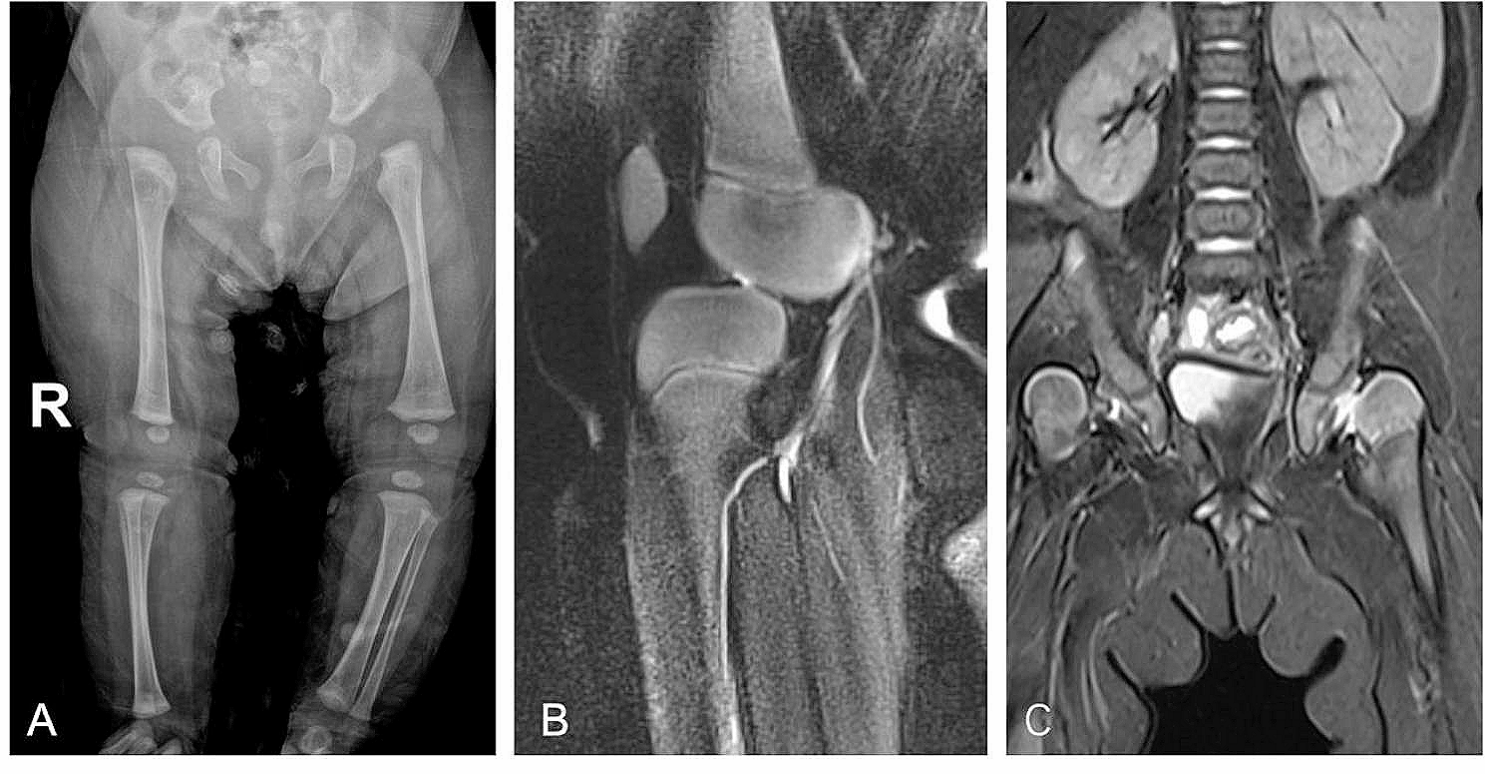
The full-length X-ray of the lower extremity revealed subluxation of the right knee joint, anterior shifting of the tibia, and external rotation (A). MRI of the right knee displayed significant anterior movement of the tibia in the right knee joint and a low and flat anterior cruciate ligament (B). MRI of the hip joint demonstrated superficial and flat bilateral acetabular development, with the bilateral femoral heads fully detached and displaced outward from the acetabular fossa (C)
